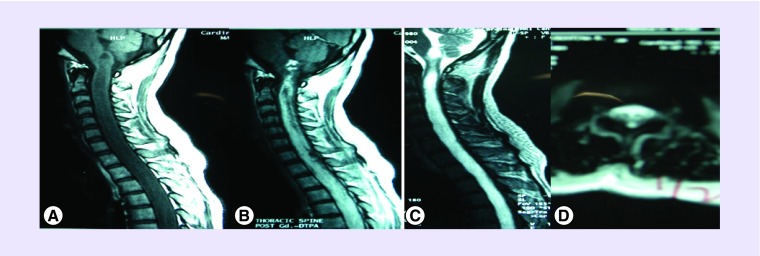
MRI findings of the patient. (A) MRI midsagittal T1-weighted image of the cervicomedullary junction and thoracic spine showing a hypointense long segment with expansile mass with a maximal diameter of 1.7 cm measured at the lower cervical region. (B) MRI with intense enhancement after administration of gadolinium. (C) T2-weighted image showing the intramedullary mass with slight heterogenous signal intensity from the level of the medulla down to the (D) axial cut section of the conus medullaris.
